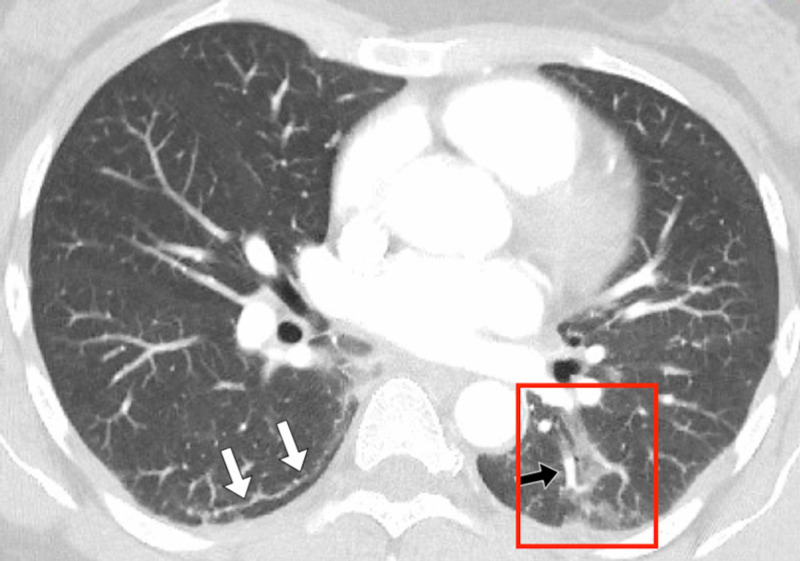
Patient 2 axial CT An axial computed tomography (CT) image in lung windows was taken at the level of the hila. The image shows a curvilinear subpleural reticular line (white arrows), vascular engorgement, and patchy peripheral and posterior ground glass opacification (red box).
